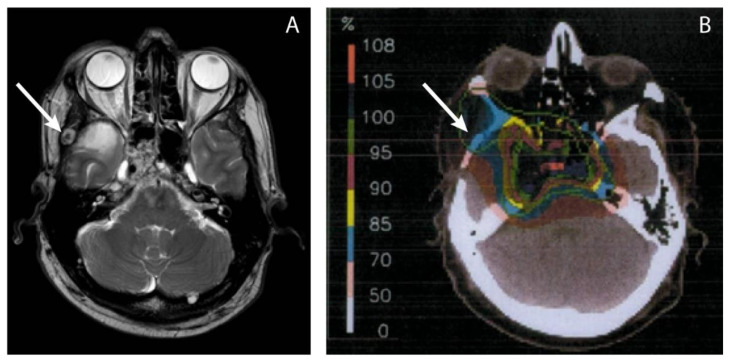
The delivered dose in the area where a metastasis was later found, was visually estimated using MRI images of the ectopic recurrence (A) and the radiotherapy dose plans (B). The arrow points toward the recurrence (A) and the estimated location on the dose plan (B), which is in the 70% isodose area, according to the isodose scale. With the prescribed dose of 100% being 74 GyRBE, the estimated dose to the region of local recurrence was 0.7 × 74 GyRBE = 51.8 GyRBE.
